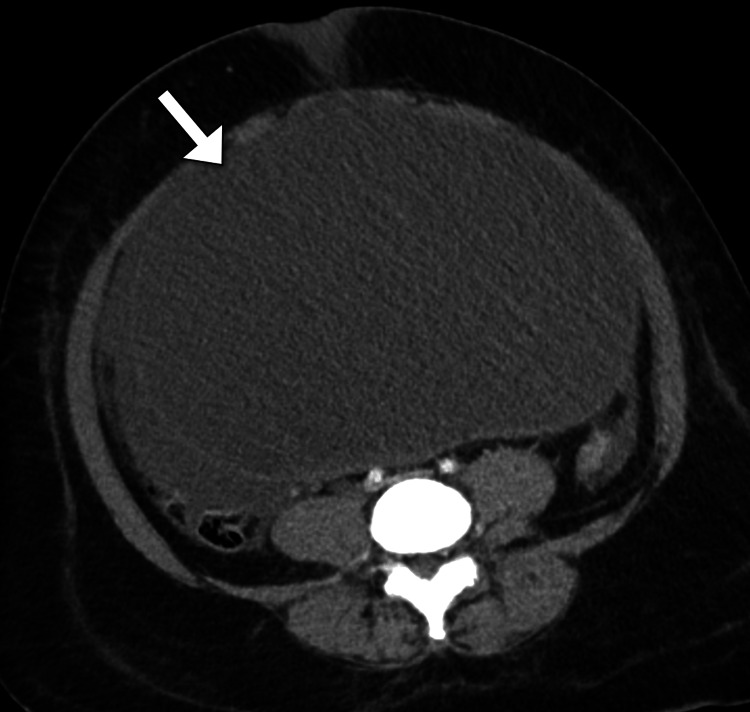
Axial abdominal CT image demonstrates a large unilocular cystic lesion (arrow) occupying most of the abdominal cavity. CT: computed tomography
